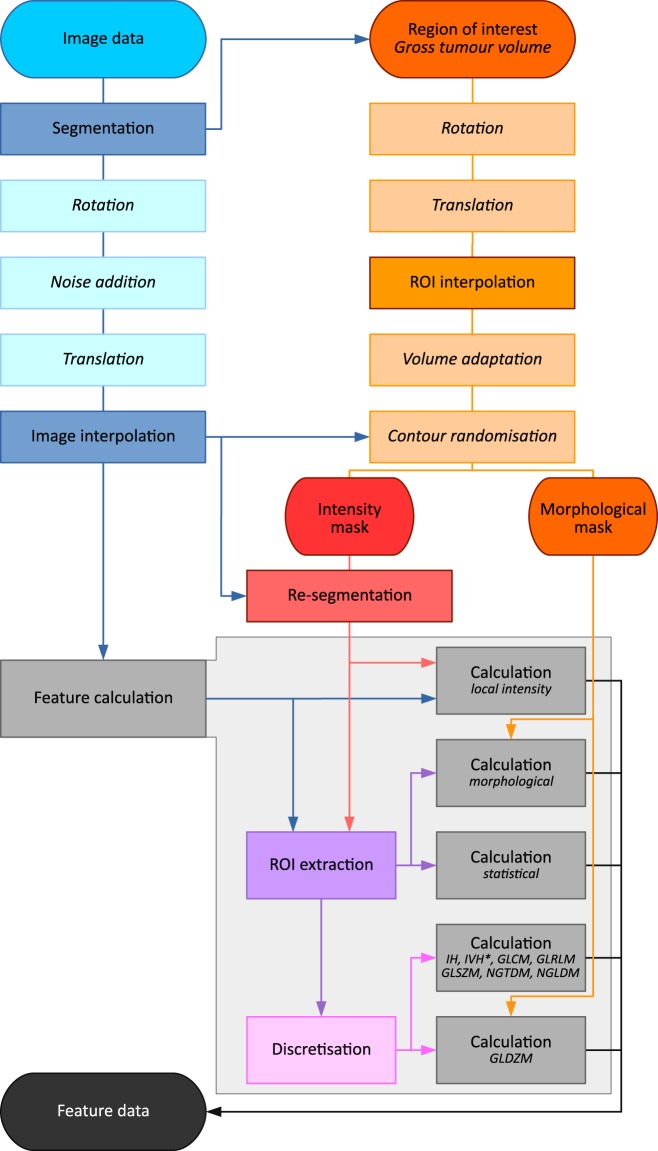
Image processing scheme with perturbations. A computed tomography (CT) image and a segmented gross tumour volume (GTV) are used as the input image data and the region of interest (ROI) respectively. The CT and ROI are processed to compute image features. Rotation, translation, noise addition, volume adaptation and contour randomisation are optional perturbation steps. Other image processing steps are detailed in the documentation of the image biomarker standardisation initiative (IBSI)^29^. IH: intensity histogram; IVH: intensity-volume histogram; GLCM: grey level co-occurrence matrix; GLRLM: grey level run length matrix; GLSZM: grey level size zone matrix; GLDZM: grey level distance zone matrix; NGDTM: neighbourhood grey tone difference matrix; NGLDM: neighbouring grey level dependence matrix. This figure is based on the image processing scheme in the IBSI document.
